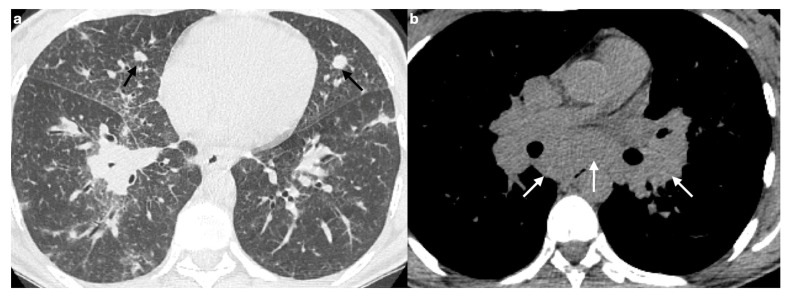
Sarcoidosis-like reaction is indistinguishable from sarcoidosis and is related to initiation of an offending drug. (a) CT scan shows bilateral parenchymal nodules (black arrows) and (b) the enlargement of hilar and mediastinal lymph nodes (white arrows) after the initiation of TNF-*α* antagonists.
